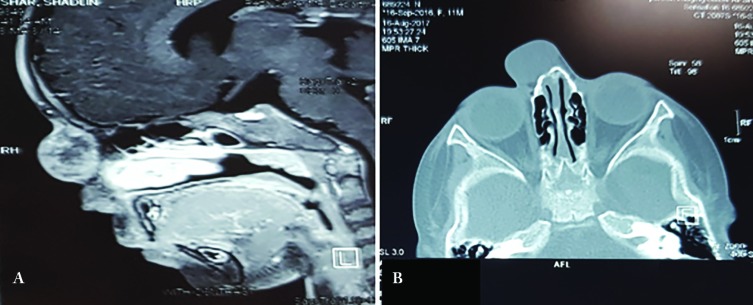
A: MRI of the mass. B: CT scan of the mass
